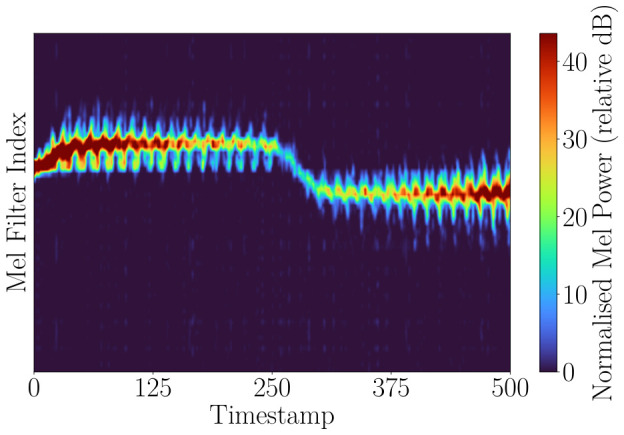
Global mel spectrogram computed from the full Doppler spectrum for a walking human. The dominant torso return is clearly visible; however, finer limb-induced micro-Doppler components are less distinguishable due to the global nature of the representation, spectral smoothing introduced by the mel transformation, and the underlying Doppler resolution. This representation provides an overall motion overview but does not explicitly separate individual motion components.
